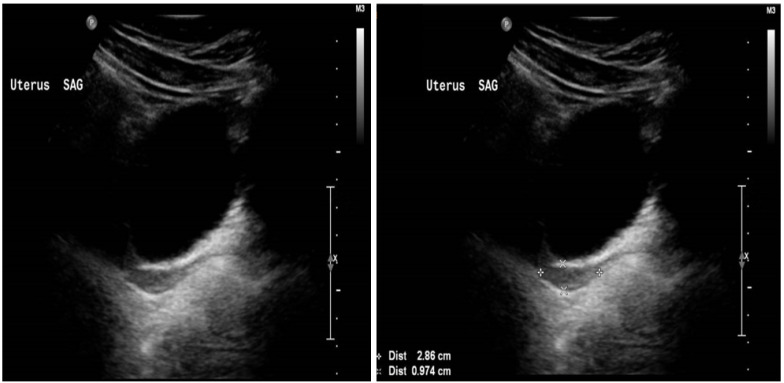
Ultrasound of the uterus at presentation when the patient aged 15 years.
